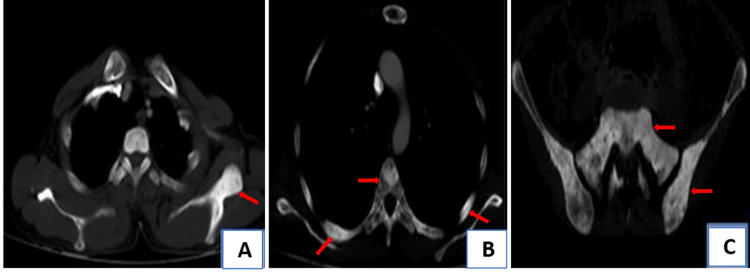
CT images in bone window setting Multiple mixed (sclerotic, lytic), mildly expansile lesions (red arrows) were seen in the left scapula (3A), bilateral ribs, and vertebral body (3B), sacrum and pelvic bones (3C).
